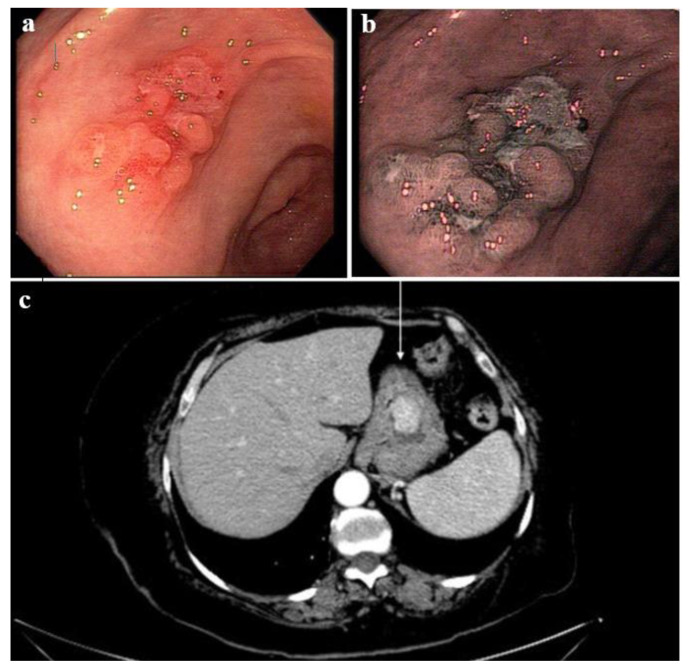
(a,b) Upper digestive endoscopy demonstrating gastric lesions compatible with MiNEN in one patient (a) the hyperemic surface of the MiNEN, associated with a central depression; (b) chromoendoscopy with magnification showing visible subepithelial capillaries; (c) CT scan showing gastric MiNEN in the gastric body of a patient.
